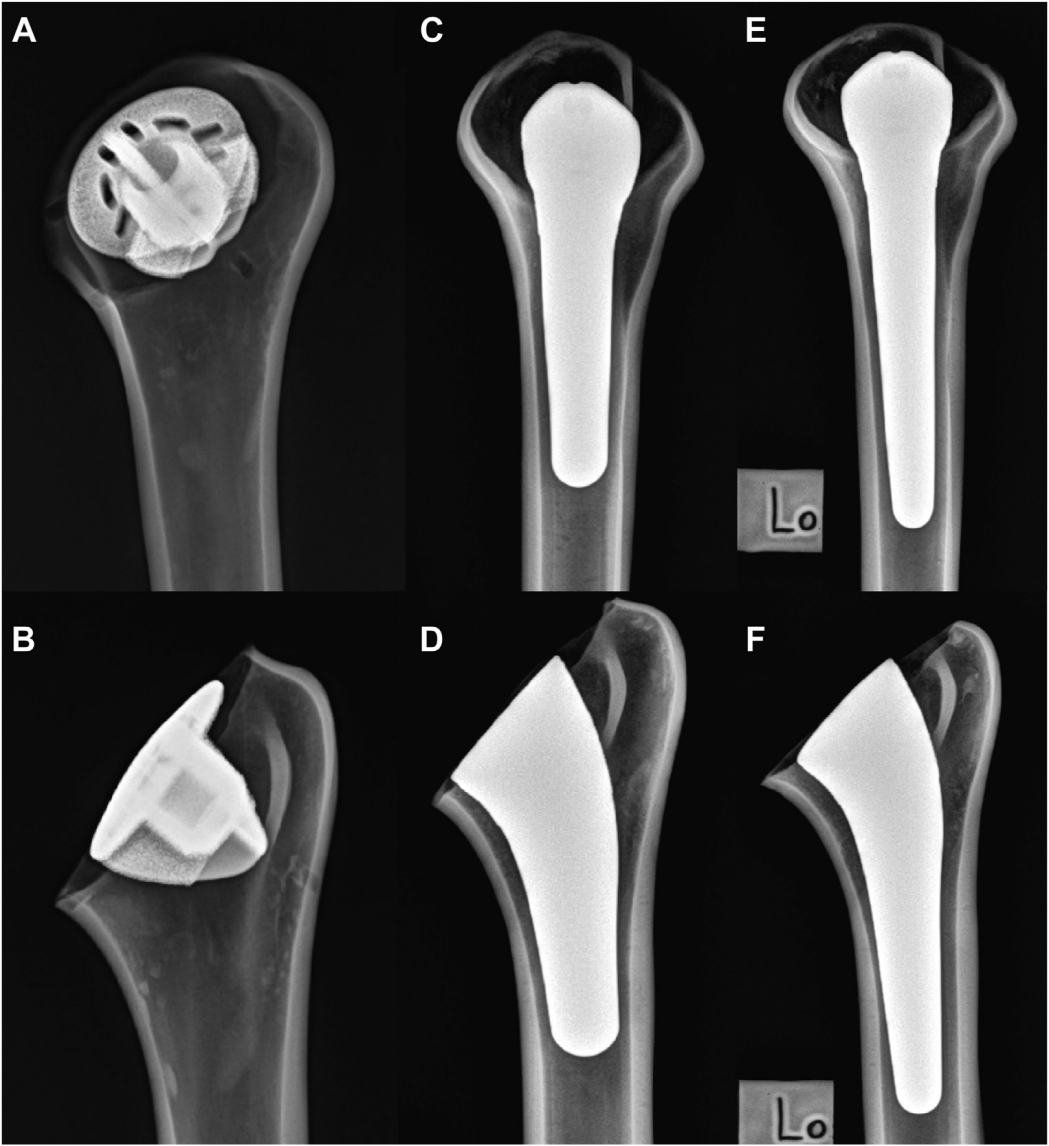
Lateral and anteroposterior radiographs of stemless (A, B), short-stem (C, D), and long-stem (E, F) implants.
